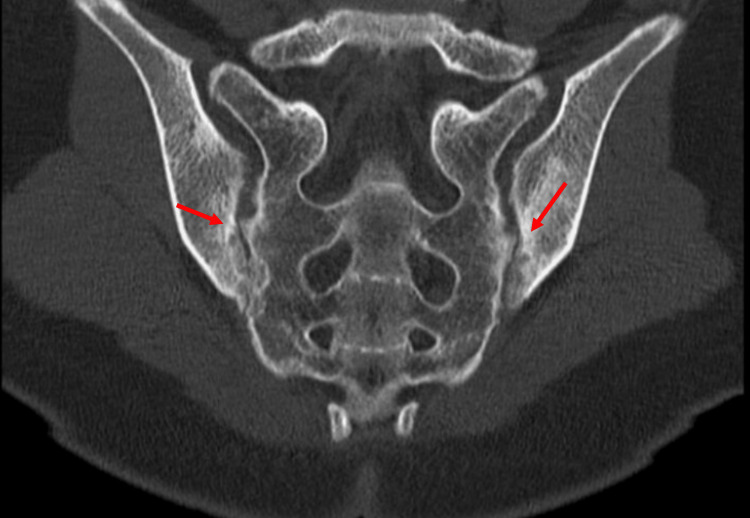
Computed tomography of the sacroiliac joint The arrow shows roughened and sclerotic joint surfaces, several tiny hypodense foci beneath the joint surfaces, and narrowing of the bilateral joint spaces
